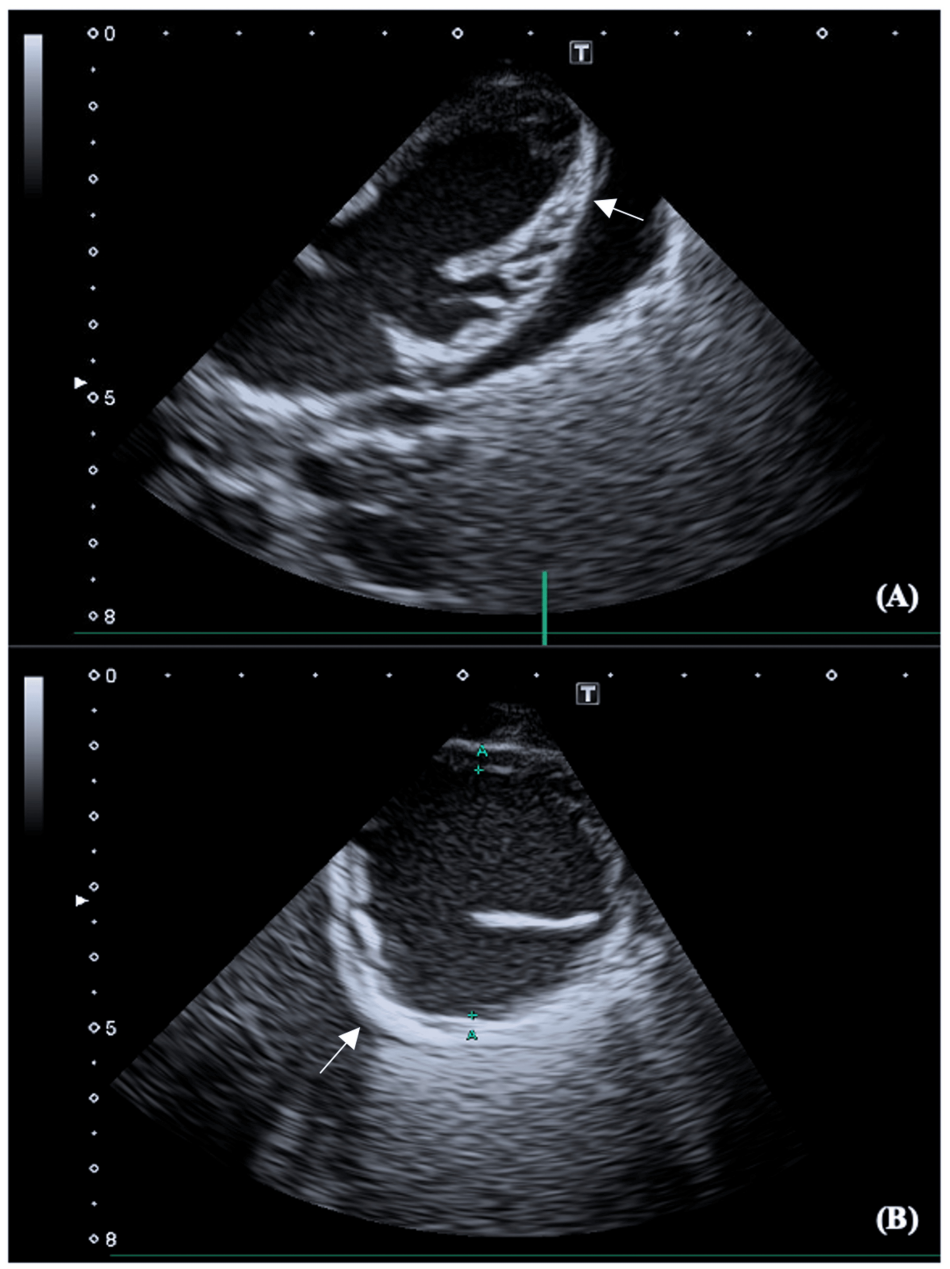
Two-dimensional echocardiogram at 50 days of life Subcostal window view (A) and parasternal short-axis view (B) showing diffuse hyperechoic areas (arrows) in the basal lateral wall. This finding is clinically significant as it may indicate evolving dystrophic calcification and irreversible myocardial injury
